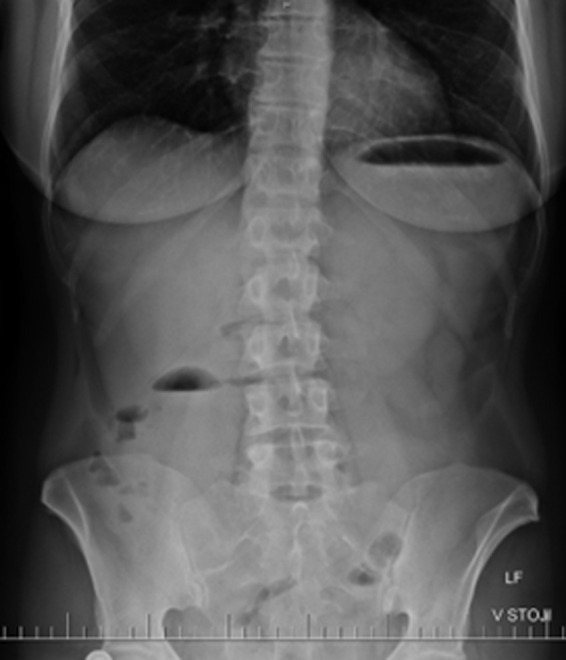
plain abdominal radiography demonstrated small bowel air-fluid levels predominantly localized to the right mesogastric region, without evidence of pneumoperitoneum
